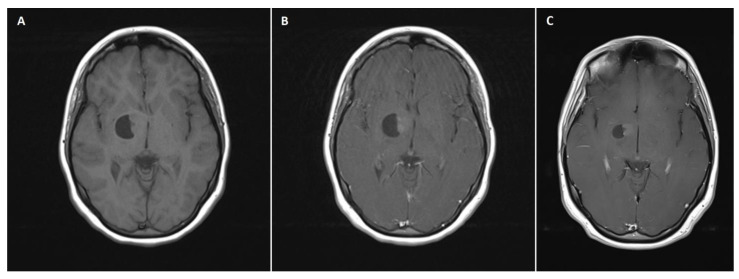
A 14-year-old girl with right-sided thalamic ganglioglioma. (A) A preoperative axial non-contrast T1 MRI scan showing an isodose solid mass located within the right thalamus with an adherent cystic component, causing a mass effect and slight midline shift. (B) The preoperative axial postcontrast T1 MRI scan revealed a heterogeneous solid mass enhancement. (C) A postoperative axial postcontrast T1 MRI scan reflecting subtotal excision of the tumor, with reduced size of the cystic component, relieved mass effect, and no evident midline shift.
